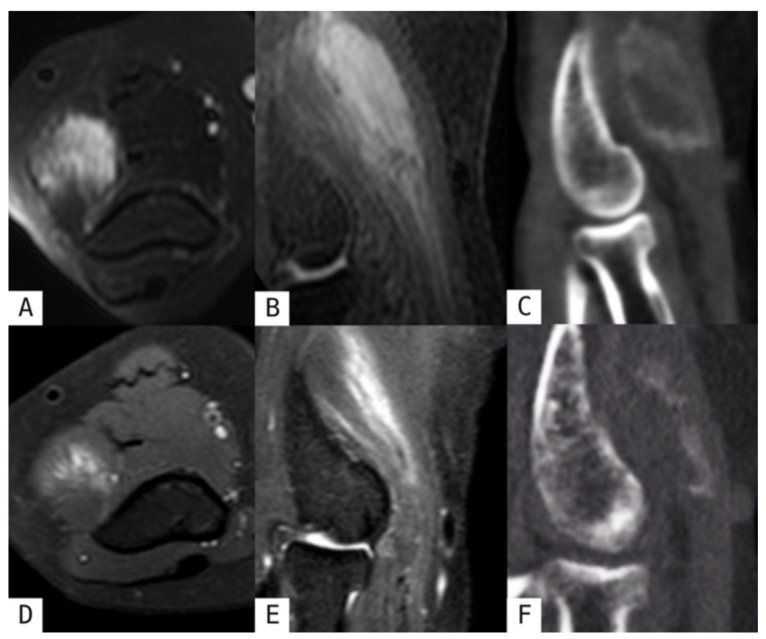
Elbow myositis ossificans before and after corticosteroid treatment. A 65-year-old woman presented with two months of post-traumatic right elbow pain. Axial T2-weighted FS (A) and sagittal STIR (B) sequences demonstrated marked diffuse oedema (“striated pattern”) with enlargement of the distal brachioradialis muscle and its myotendinous junction. Multiplanar CT sagittal reconstruction (C) revealed peripheral eccentric calcifications. One month after corticosteroid therapy, follow-up axial PD (D) and sagittal STIR (E) sequences revealed reduced oedema and lesion size, while sagittal 2D MPR reconstruction CT (F) showed regression of the peripheral calcifications.
